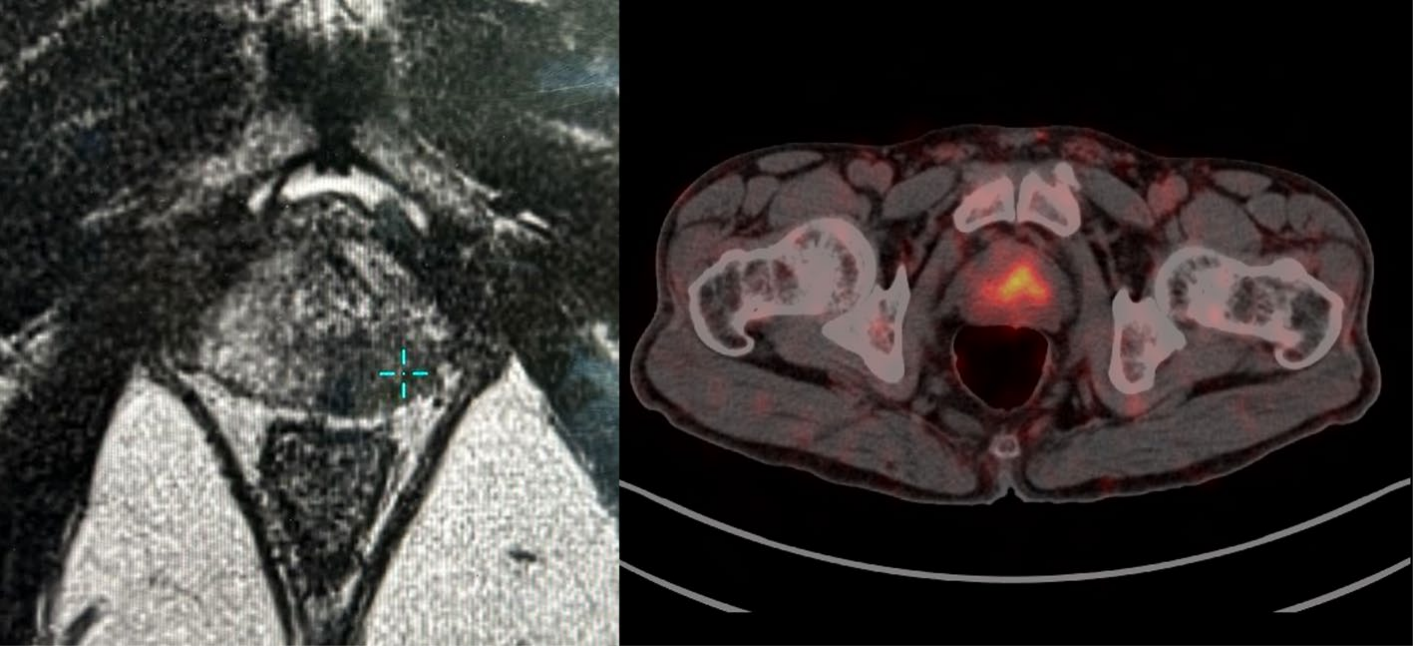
Patient with PIRADS 4 lesion on multi-parametric MRI showing focal tracer uptake is noted in the central zone of the left mid prostate (SUVmax 6.8). Mild inhomogeneous tracer uptake is noted in rest of the prostate gland. Biopsy revealed adenocarcinoma Gleason 3 + 4
